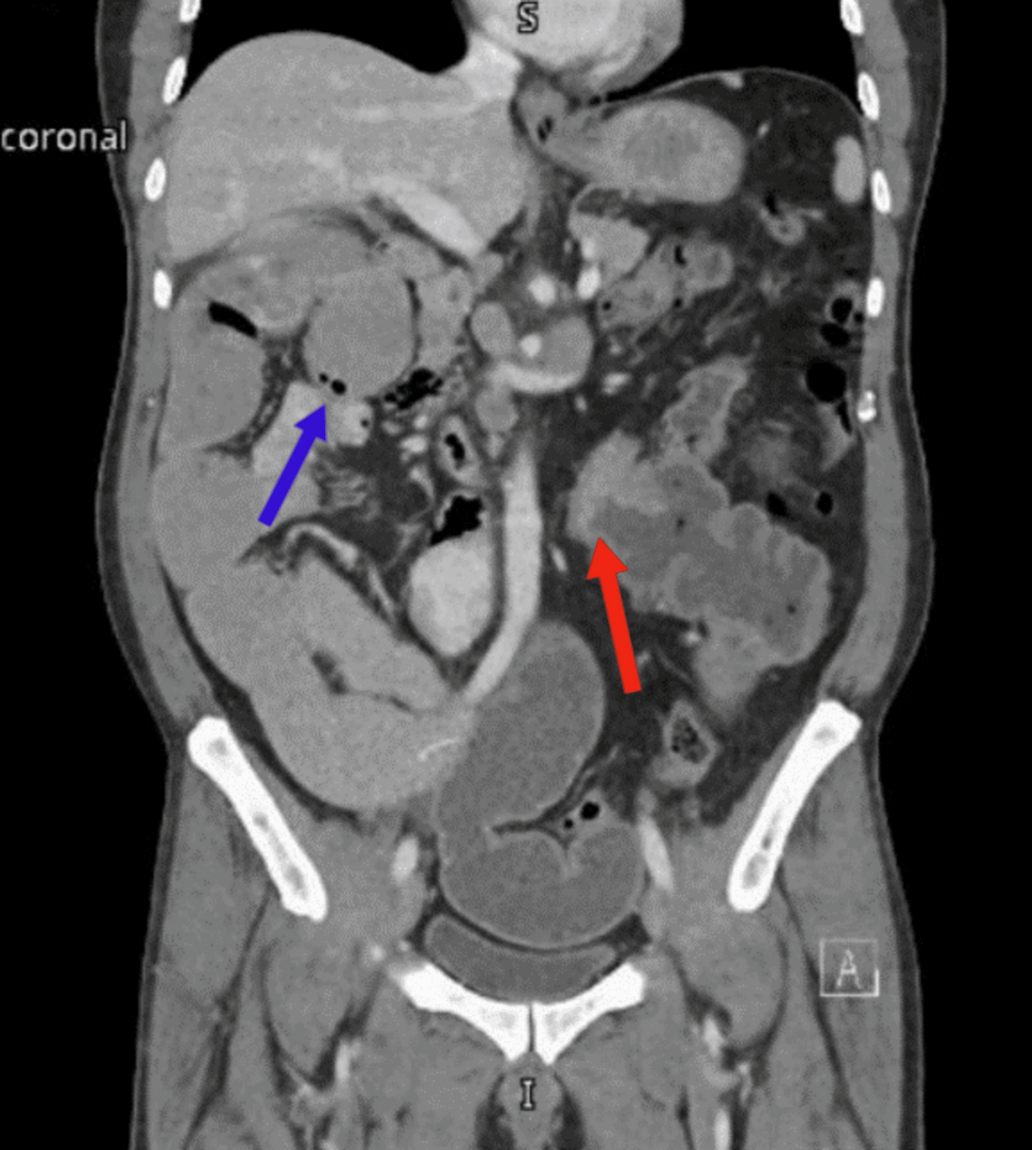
Coronal view on CT of the abdomen showing the obstructing ascending colon tumor (red arrow) on the left side of the abdomen and the distended small bowel loops on the right side of the abdomen including the duodenojejunal junction (blue arrow). CT: computed tomography
